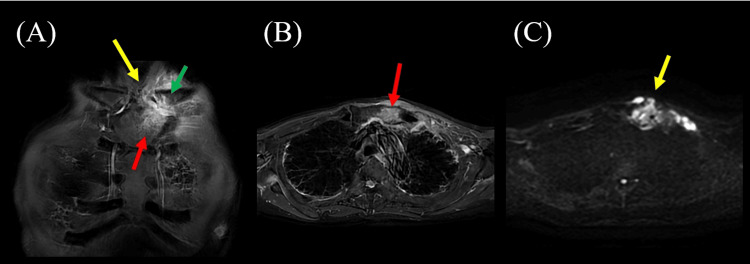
Results of the chest magnetic resonance imaging (MRI) two months after stroke. (A, B, C) Chest MRI showing soft tissue signal around and within the left sternoclavicular joint (yellow arrows). High signal intensity is also observed in the sternum (red arrow) and clavicle (green arrow). (A) Coronal section (short τ inversion recovery [STIR]); (B) axial section (short τ inversion recovery [STIR]); (C) axial section (diffusion-weighted imaging [DWI]).
